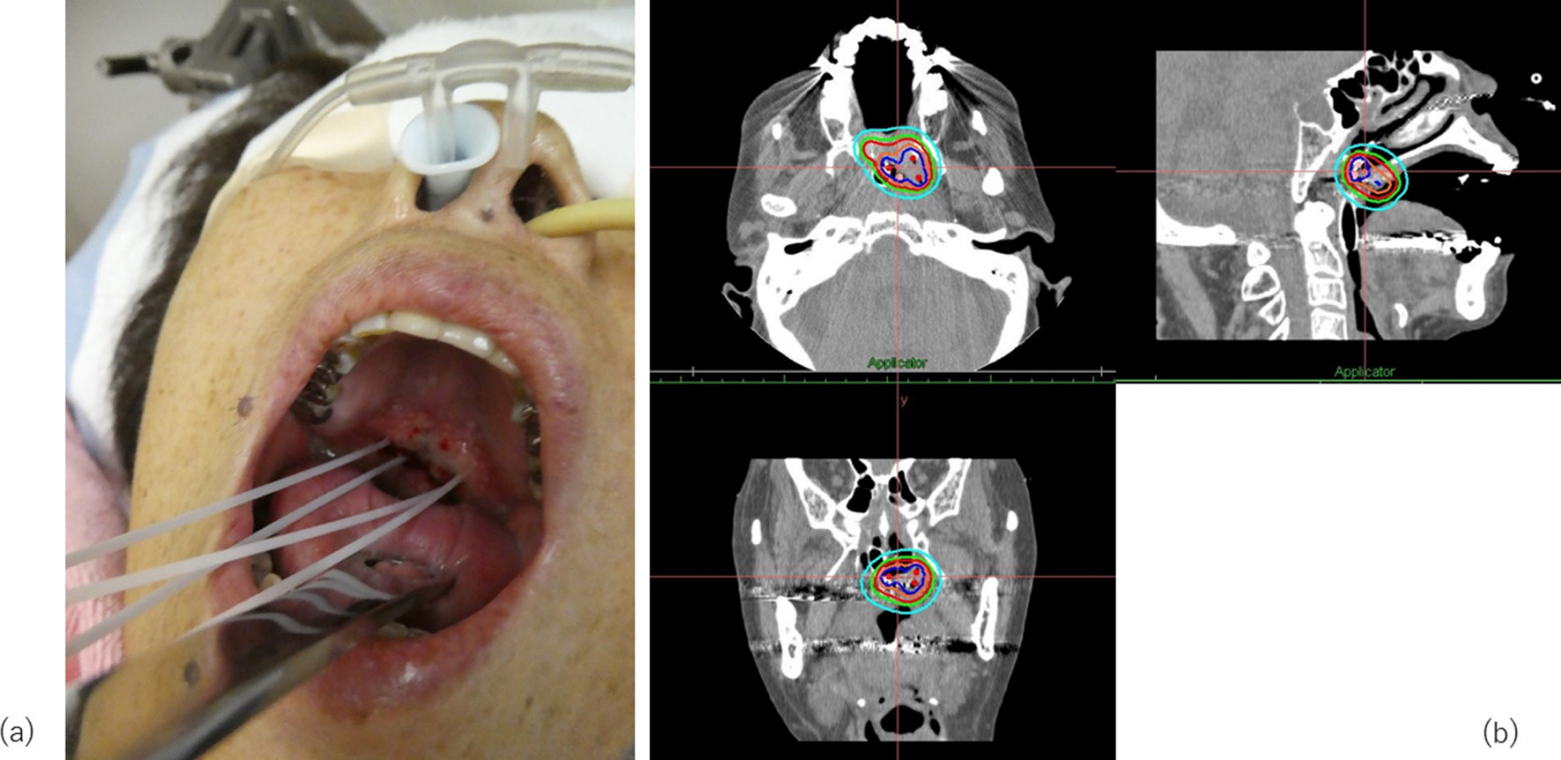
(a) Under local anesthesia and sedation, four 5 French ProGuide® sharp plastic needles (Nucletron, an Elekta company, Elekta AB, Stockholm, Sweden) were inserted transorally through the tumour. Depth of the needles was determined by CT image. (b) Isodose distribution of the interstitial implant with the red and blue line representing the 100 and 200% isodose, respectively.
